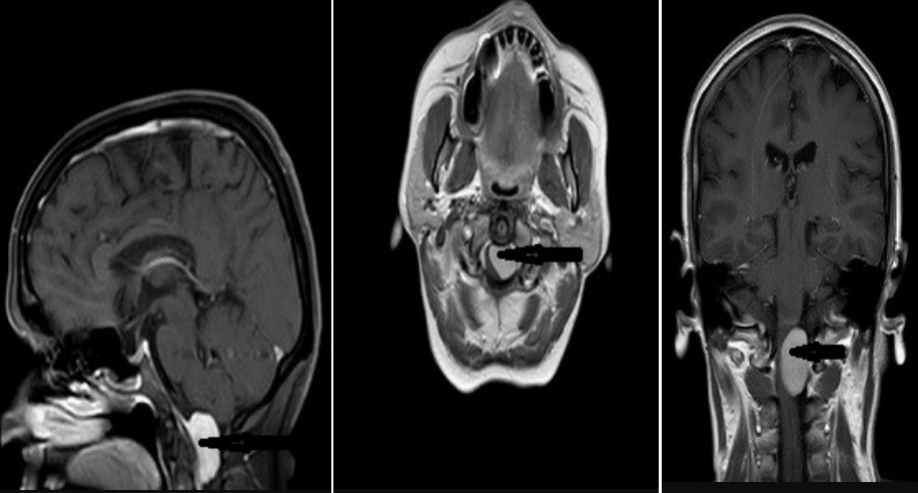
54 years old female patient pre-op MRI images and back arrow indicates tumor
